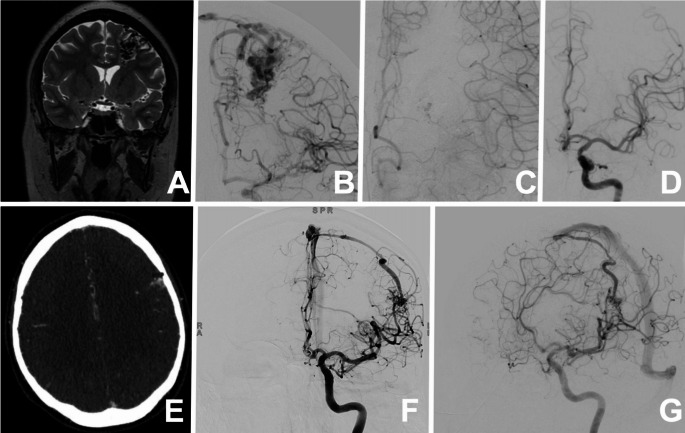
(A) Magnetic resonance imaging (MRI) demonstrating an arteriovenous malformation (AVM) located in the perirolandic territory. (B) Digital subtraction angiography (DSA) of the same lesion, showing arterial feeders arising from branches of the left middle cerebral artery and anterior cerebral artery. (C) Postoperative angiography revealing a minimal deep residual lesion adjacent to the internal capsule. (D) Six-month follow-up angiography demonstrating spontaneous thrombosis of the residual nidus. (E) Second case: non-contrast CT showing a small AVM in contact with proximal branches of the ipsilateral middle cerebral artery, which provide its arterial supply. (F) Corresponding angiographic image of the second case. (G) Postoperative angiography showing a minimal residual AVM, intentionally left due to transient loss of neurophysiological potentials during temporary clamping of the afferent vessels; the patient is currently under radiological follow-up with no evidence of new hemorrhages
